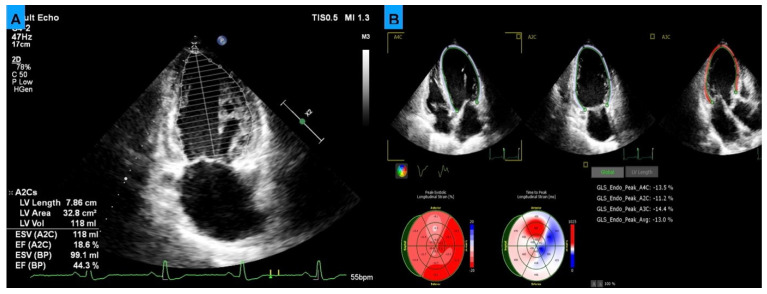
Reference transthoracic echocardiography (TTE) parameters. (A) Left ventricular ejection fraction (EF) calculated using the biplane Simpson’s method from apical four-chamber and two-chamber views. (B) Global longitudinal strain (GLS) obtained by speckle-tracking echocardiography, showing strain curves and a bull’s-eye plot for quantitative assessment of left ventricular systolic function.
